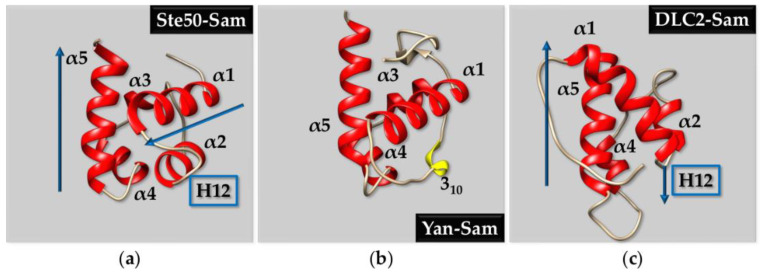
Sam domain fold. (a) NMR structure of the *Saccharomyces cerevisiae* Ste50-Sam domain (PDB entry 1Z1V [79]). The α-helices (α1 from V37 to L48, α2 from P55 to E62, α3 from L70 to E72, α4 from L75 to L81 and α5 from L86 to D101) are reported in red. The reciprocal orientation of α5 and the helical H12 hairpin (including α1, α2 and connecting loop) is indicated by blue arrows. (b) X-ray structure of the Sam domain mutant A86R of Yan protein (PDB entry 1SV4 [78], chain A). The α-helices (α1 from R56 to F70, α3 from G84 to L89, α4 from R92 to R98 and α5 from G103 to H118) are reported in red, whereas the 3_10_ helix, encompassing residues from F77 to F80, is reported in yellow. (c) NMR structure of the DLC2-Sam domain (PDB entry 2H80 [80], conformer n.1 of the NMR ensemble). The α-helices (α1 from Q17 to A30, α2 from P34 to Q38, α3 from I49 to N54 and α4 from V64 to A78) are shown in red. The first 5 N-terminal residues have been removed to better visualize the H12 helical hairpin. The mutual orientation of α5 with respect to the H12 helical hairpin is indicated by blue arrows.
